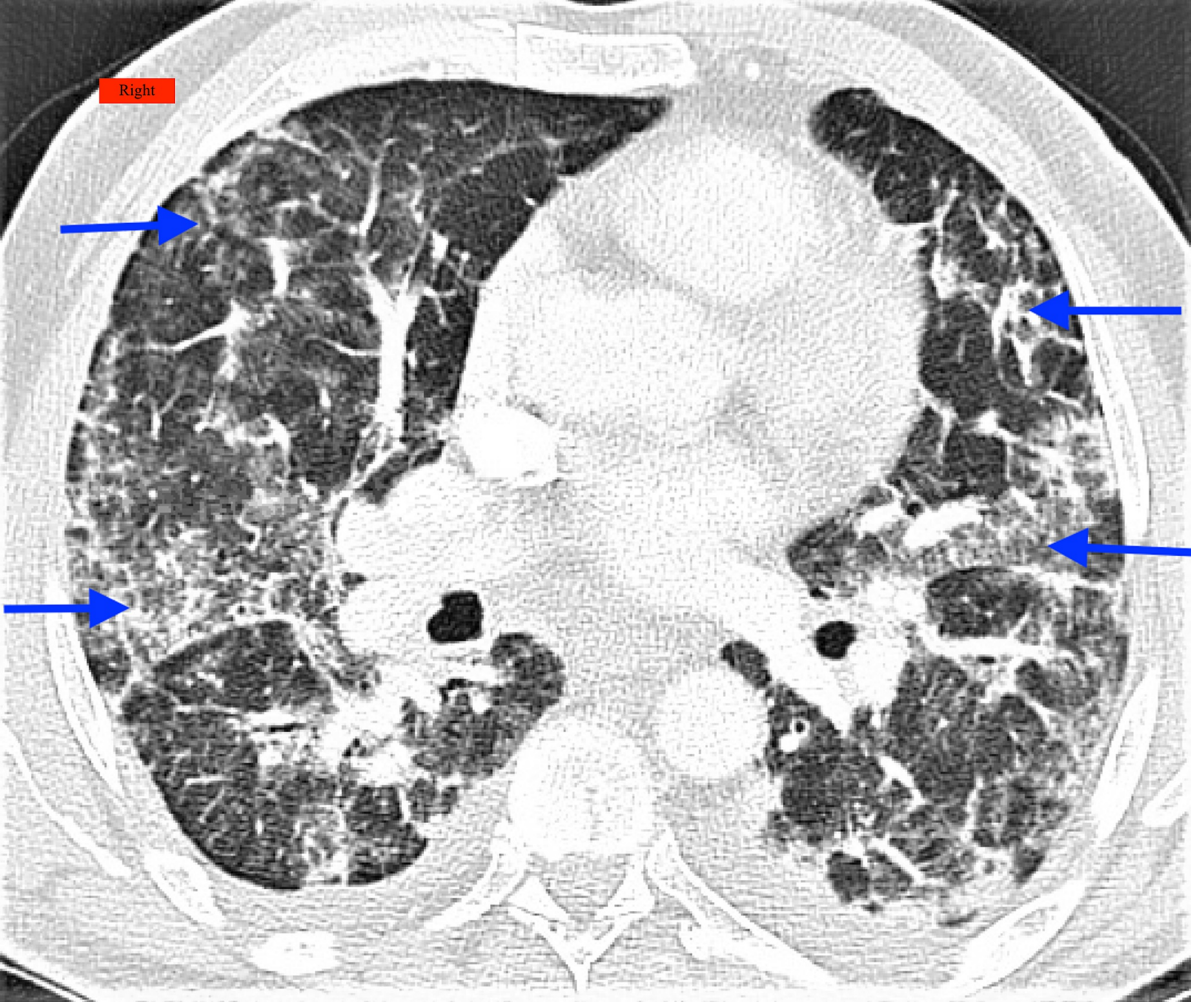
CT scan of the chest showing diffuse interstitial and airspace opacities bilaterally along with small bilateral pleural effusion Right: Indicates the right side of the patient; CT: Computed Tomography
